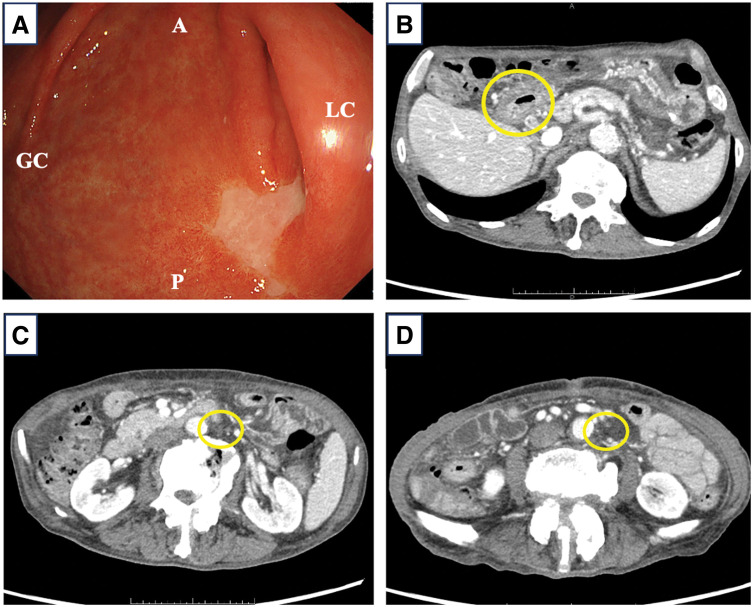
Upper gastrointestinal endoscopic and contrast-enhanced CT findings after chemotherapy. (A) Upper gastrointestinal endoscopy showing the tumor shrinkage and white scar. (B) Contrast-enhanced CT showing the main tumor shrinking. (C, D) The size of the para-aortic lymph nodes nos. 16b1 (C) and 16b2 (D) significantly decreased (circles). A, anterior wall; GC, greater curvature; LC, lesser curvature; P, posterior wall
